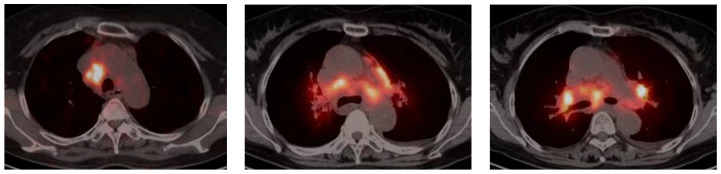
PET/CT images of pulmonary sarcoidosis.
